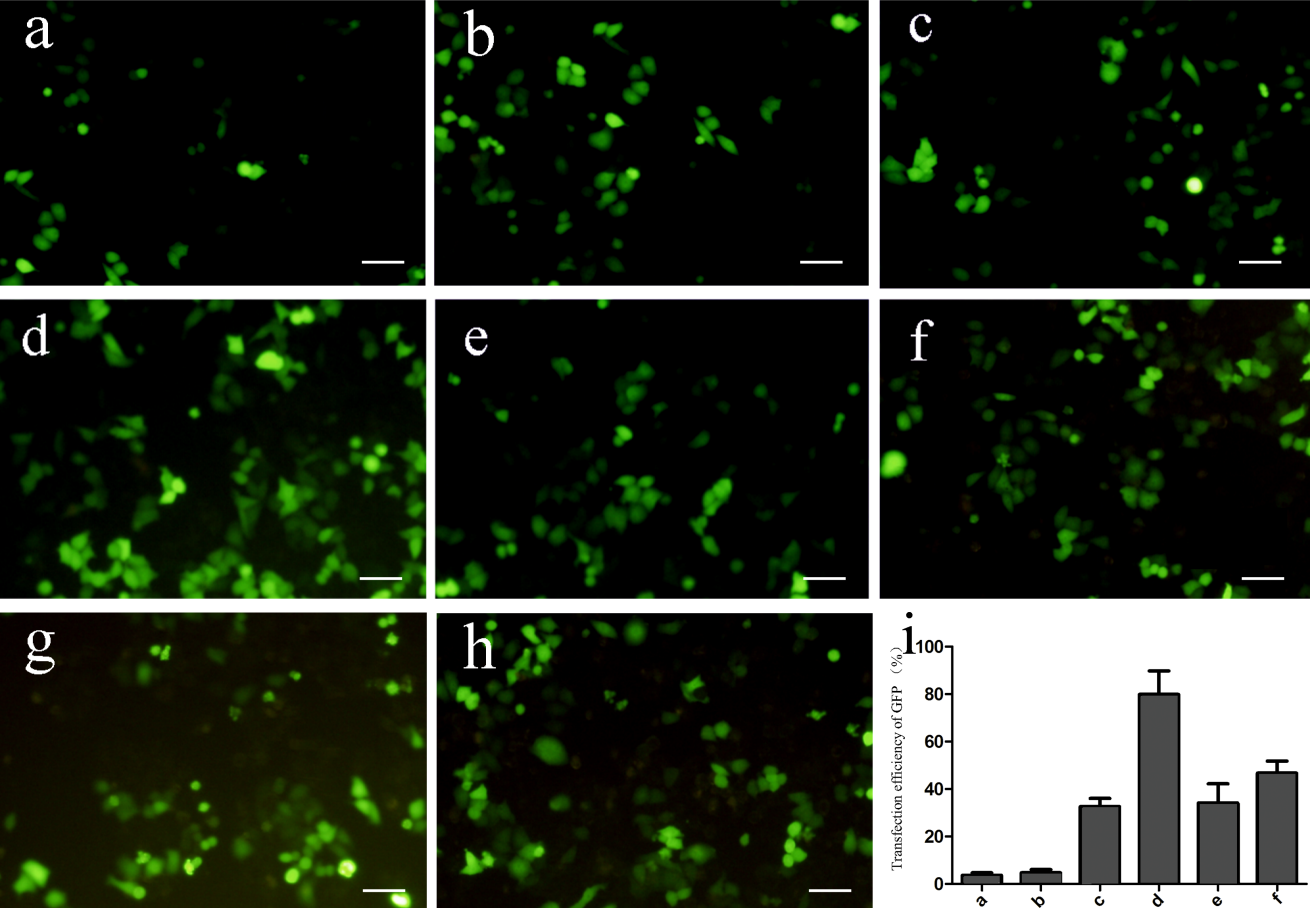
Differences in GFP transfection efficiencies of NBs and liposomes (×200) and GFP transfection with or without ultrasound irradiation (×200). a. 8 μg of plasmid; b. 8 μg of plasmid and ultrasound irradiation; c. NBs and 8 μg of plasmid; d. NBs, 8 μg of plasmid and ultrasound irradiation; e. liposomes and 8 μg of plasmid; f. liposomes, 8 μg of plasmid and ultrasound irradiation; g. NBs and 4 μg of plasmid; h. liposomes and 4 μg of plasmid; i. FCM assessment of GFP expression in transfected cells with or without ultrasound irradiation; expression in group d is significantly higher than in other groups (P*<0.05).
